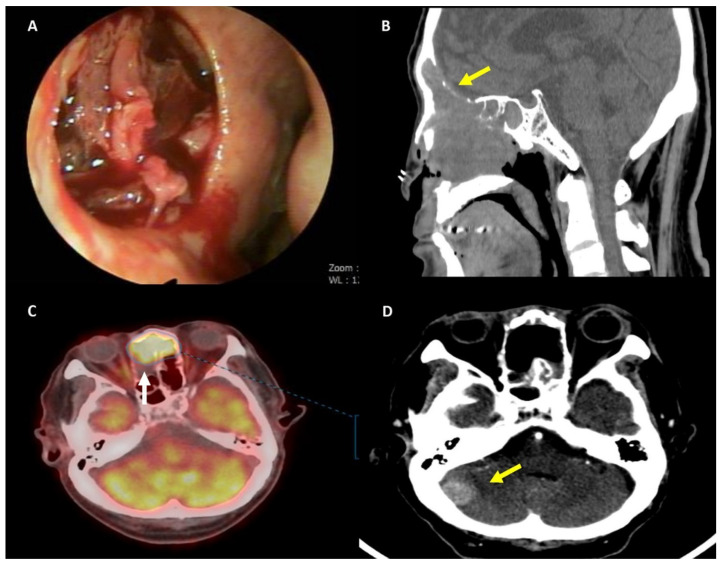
A 65-year-old male was referred to the department of otorhinolaryngology with epistaxis and right nasal obstruction for two months. His medical history revealed diabetes mellitus, hypertension, and pulmonary tuberculosis. (A) Physical examination demonstrated an easy-bleeding tumor in the right nasal cavity and bloody crust with an ulcerative lesion at the anterior septum; (B) The initial computed tomography (CT) showed a 4.5 cm × 3.4 cm × 4.8 cm soft tissue lesion infiltrating the right ethmoid and maxillary sinuses with destruction at the frontal sinus posterior wall and cribriform plate. Magnetic resonance imaging (MRI) showed a residual mass with destruction in the posterior wall of the frontal sinus, cribriform plate, and dura invasion with suspicious brain parenchyma (right frontal lobe) invasion; (C,D) Fluorodeoxyglucose positron emission tomography (FDG-PET) showed a residual hypermetabolic mass involving the superior portion of the nasal cavity and the anterior portion of the ethmoid sinus, with a maximum standardized uptake value (SUVmax) of 8.5. Clinical differential diagnoses included inverted papilloma with malignant transformation, lymphoma, and olfactory neuroblastoma. To confirm the diagnosis, an excisional biopsy was conducted.
